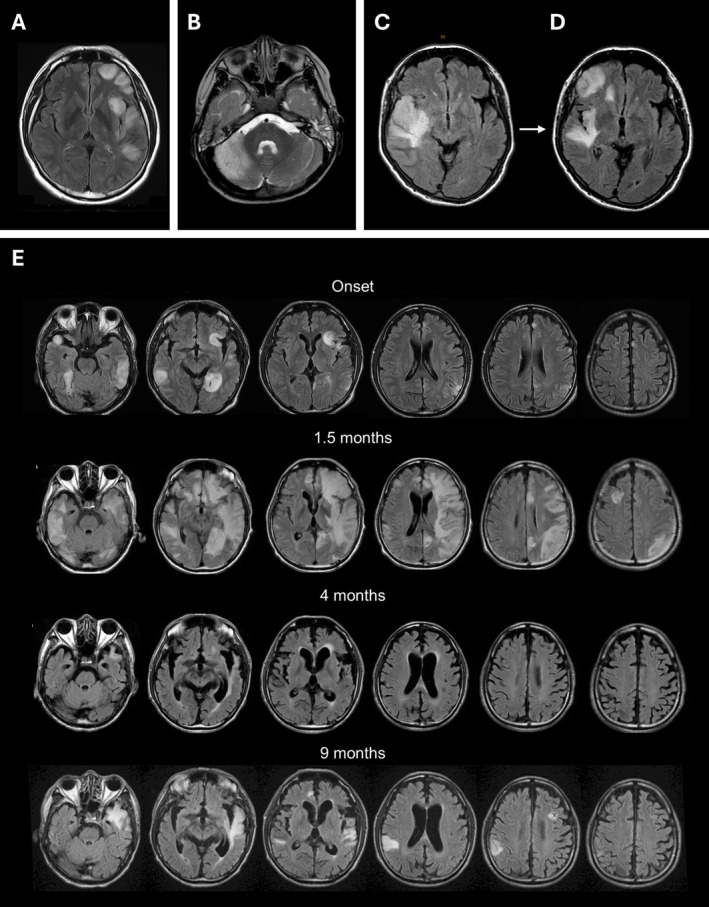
Brain magnetic resonance imaging (MRI) features and changes over time in patients with anti‐*γ*‐aminobutyric acid type A receptor (GABA_A_R) encephalitis. (A–D) Initial brain MRIs findings showing: (A) multifocal supratentorial cortico‐subcortical T2/fluid‐attenuated inversion recovery (FLAIR) hyperintense lesions in the left temporo‐fronto‐parietal lobes (patient 10); (B) upper right cerebellar hemisphere lesions with minor involvement of left cerebellar folia (patient 1), without contrast enhancement or diffusion restriction (not shown); (C) initial single cortico‐subcortical T2/FLAIR hyperintense lesion in the right temporal lobe that evolved at relapse (4 months later) into multiple right frontal lesions with re‐expansion of the prior temporal lesion (D) (patient 17). (E) Dynamic changes of brain lesions overtime during the disease course (patient 23). At disease onset, MRI showed multifocal and bilateral T2/FLAIR hyperintense lesions, which worsened during the initial episode eventually merging into confluent areas after 1.5 months. Four months after onset, most of the previous T2/FLAIR abnormalities resolved, and a diffuse brain atrophy developed, predominantly affecting bilateral fronto‐temporal lobes. Nine months from onset, the previous inflammatory lesion in the left temporal cortex enlarged, along with the appearance of new small lesions in the parietal and frontal cortices, without apparent worsening of neurological symptoms. [Color figure can be viewed at www.annalsofneurology.org]
